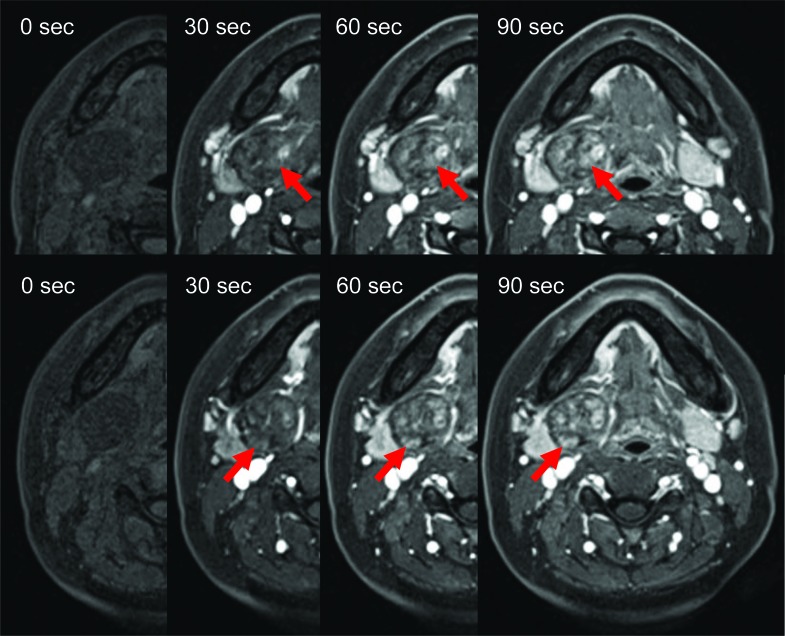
Dynamic MRI showed heterogeneous gradual increased enhancement. Regions of gradual increased enhancement were observed (red arrows).
